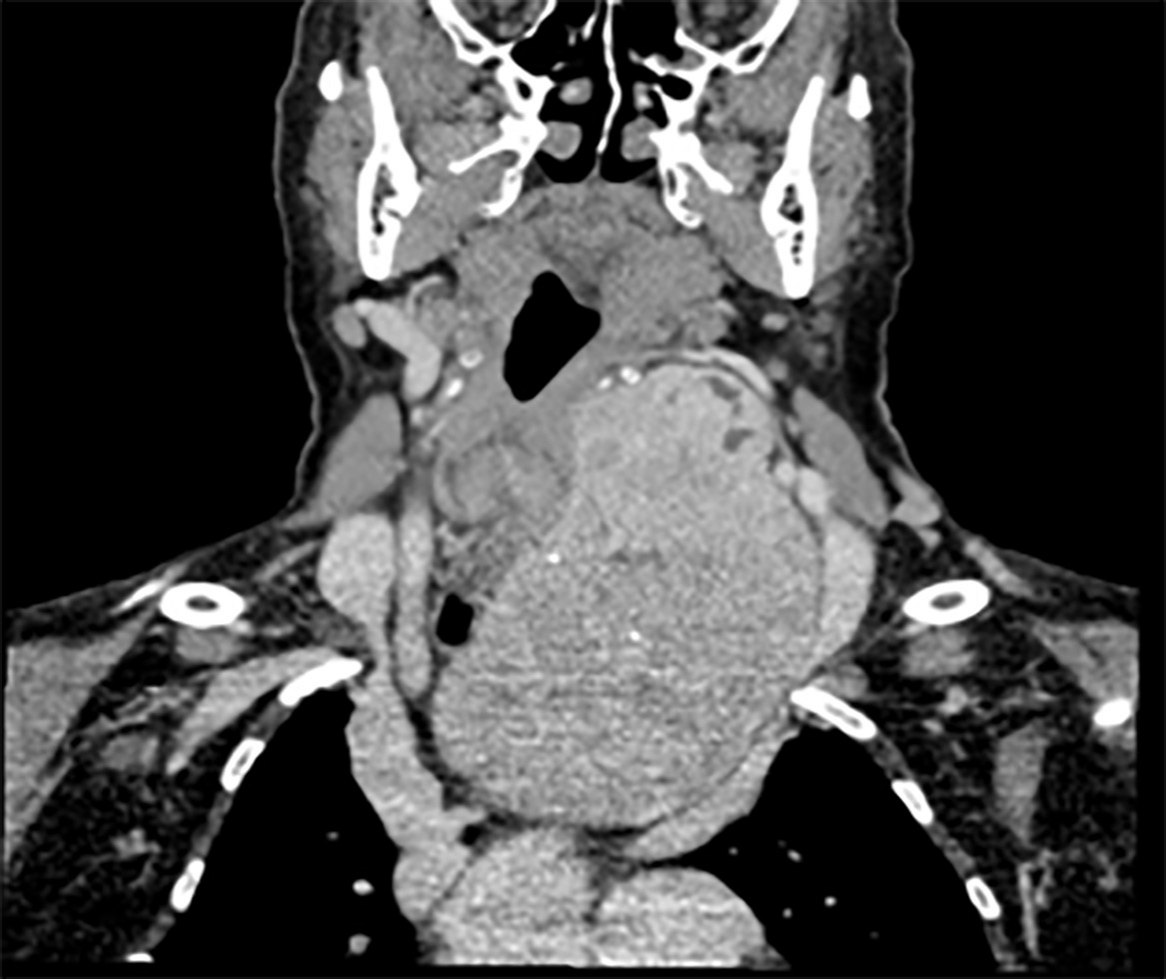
Preoperative CT of a patient with SG, showing a possible compression of the vagus nerve against the first rib.
